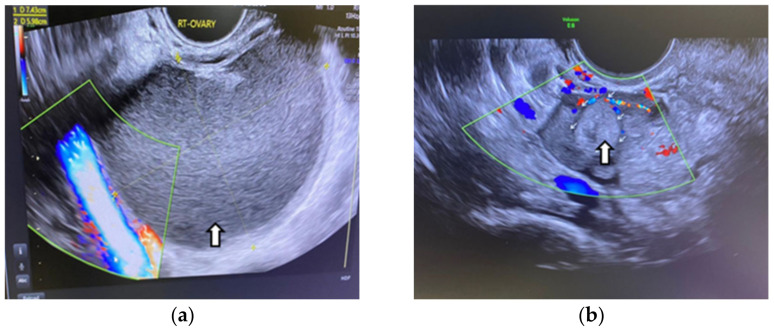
Vaginal ultrasound image of an ovarian endometrioma treated by sclerotherapy: (a) Arrow shows the original aspect of the endometrioma (b) Arrows show the endometrioma 6 months after ethanolic sclerotherapy. A considerable reduction in size can be seen.
